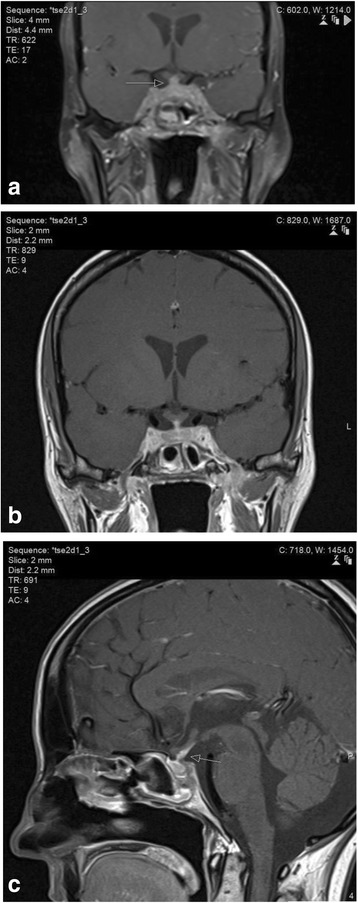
MR images of the brainstem, skull base and pituitary gland. (a) Coronal contrast-enhanced fat-saturated T1-weighted image of the central skull base demonstrates pathological enhancement of the cavernous sinus, pituitary gland and pituitary stalk (arrow). The abnormalities are non-discrete and radiologically inconsistent with a microadenoma or invasive macro adenoma of the pituitary gland. (b) Dedicated thin-section coronal contrast-enhanced T1-weighted image of the pituitary demonstrates the abnormal enhancement and thickening of the pituitary stalk as well as the cavernous sinus and pituitary parenchyma in more detail. (c) Dedicated thin-section sagittal contrast-enhanced T1-weighted image of the pituitary demonstrates the abnormal generalised thickening and enhancement of the pituitary stalk (arrow) consistent with granulomatous infundibulitis
